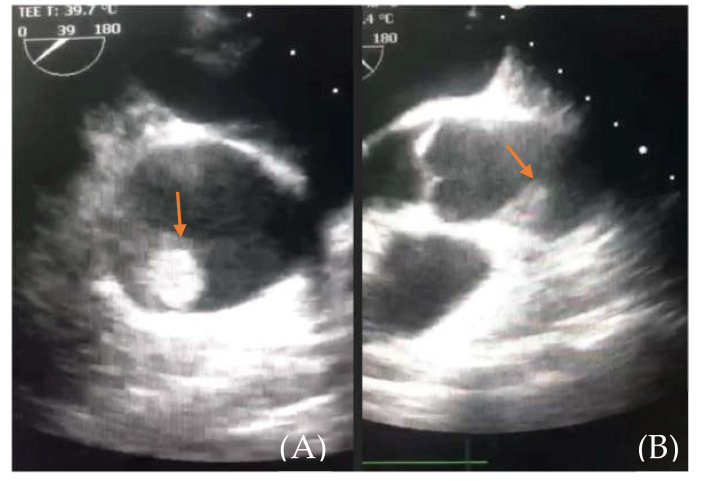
(A) (thrombus—orange arrow) and (B) (aortic root and thrombus). Emergency room transesophageal echocardiography showing the floating thrombus located in the ascending aorta.
